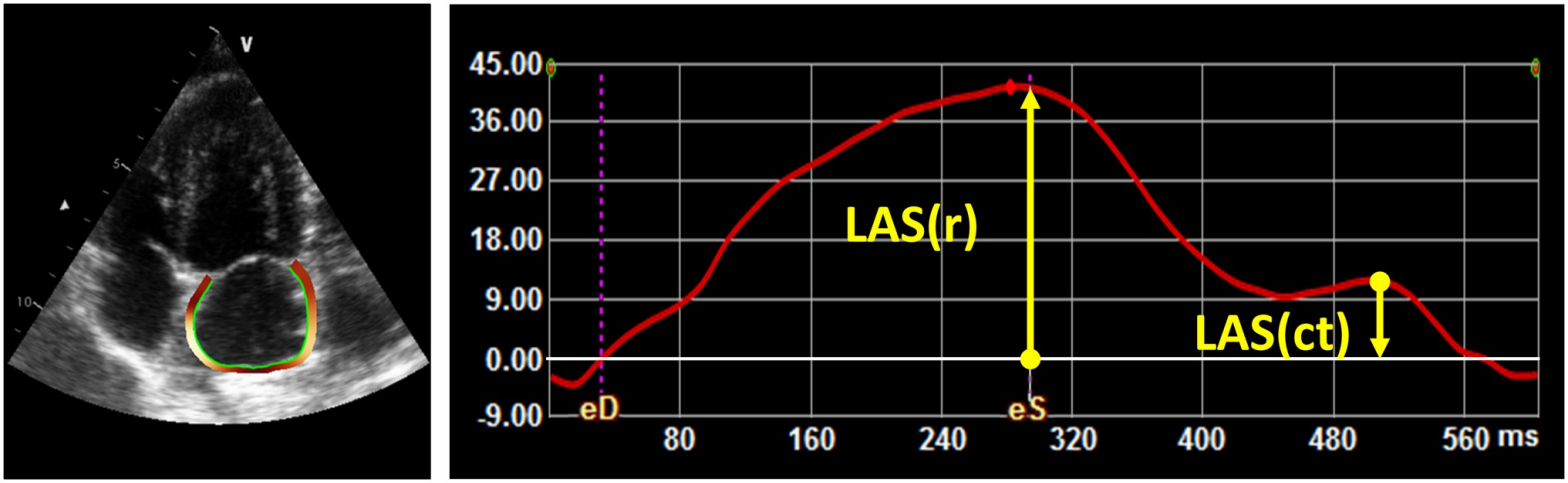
Example of LA strain assessment by 2D speckle-tracking echocardiography at baseline. Four-chamber longitudinal left atrium strain (LAS). Arrows represent reservoir (r) and contractile (ct) phase (42% and -12%, respectively).
